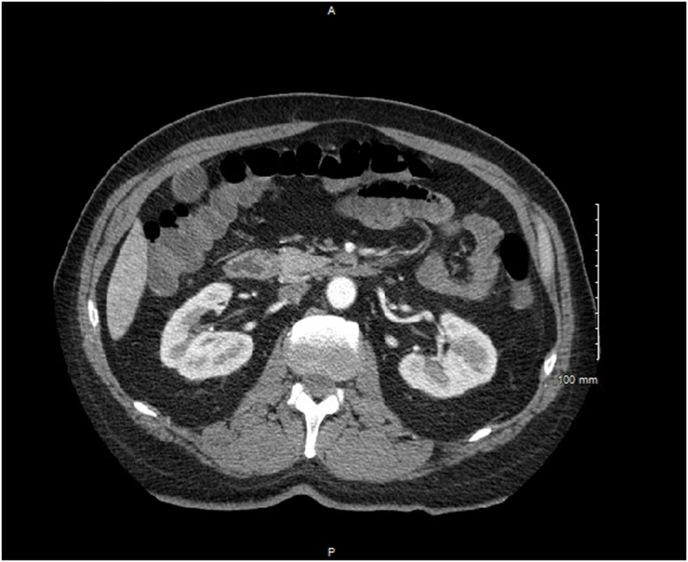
Computed Tomography Angiography of Abdomen & Pelvis with contrast displaying intraluminal fluid enhancement in the colon suggestive of viral enterocolitis. No bowel wall thickening or bowel edema reflective of inflammatory changes was noted. (Axial View).
